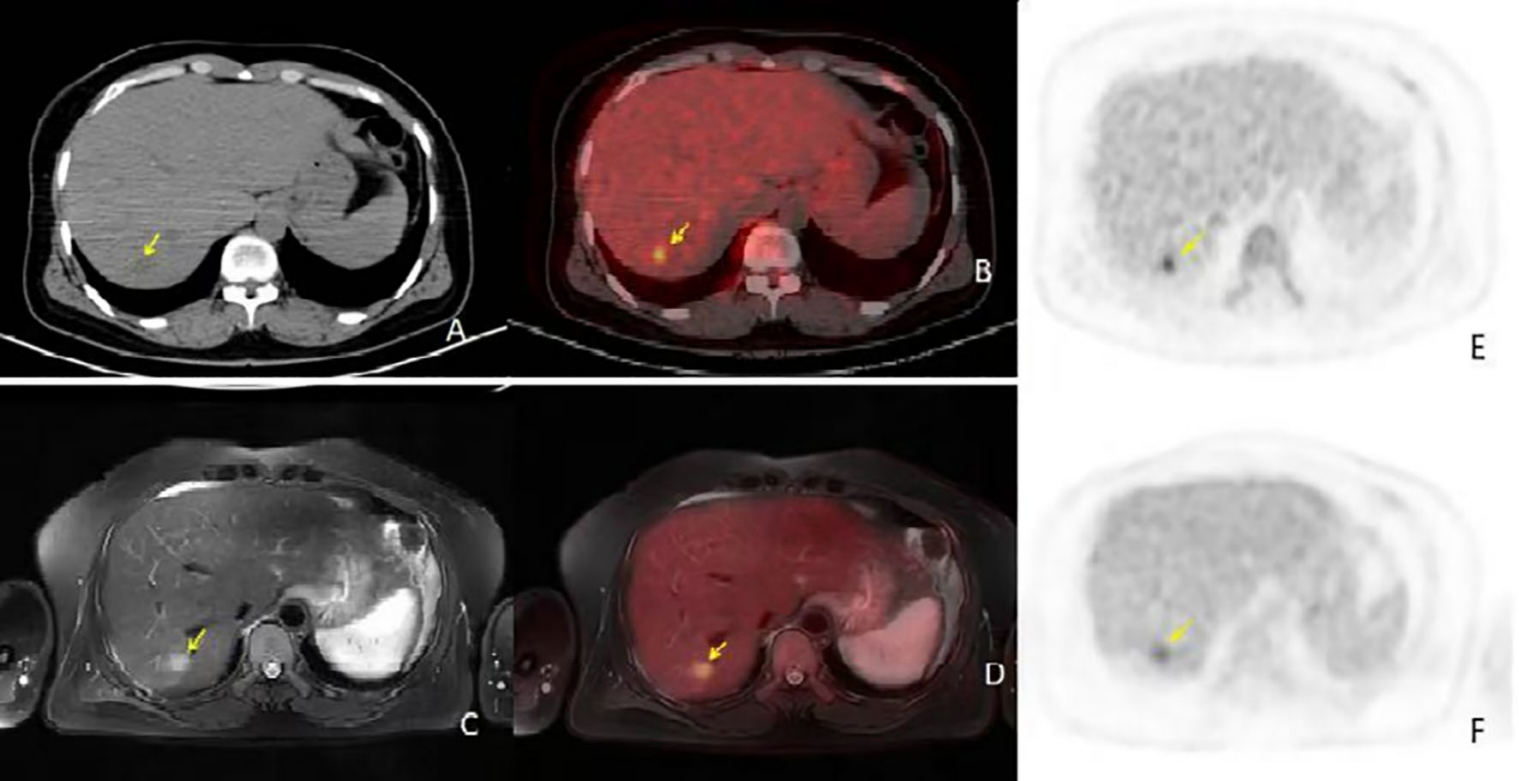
(A, B) are conventional PET/CT scan images [(A) is the CT image, (B) is the fusion image], (C, D) are PET/CT 2-hour delayed scan images [(C) is the CT image, (D) is the fusion image], and E and F are PET/MR images [(E) is the T2WI image, (F) is the fusion image]. The PET/CT lesion in the CT image shows isodensity, with no significant metabolic activity in the conventional scan. In the delayed scan, mild metabolic activity is seen, approaching the liver parenchyma, SUVmax 4.5. In the PET/MR image, the T2WI lesion shows high signal with mild metabolic activity, SUVmax 4.0. PET/MR provided a clearer diagnosis compared to PET/CT, and the PET/CT 2-hour delayed imaging offered a clearer diagnosis compared to conventional imaging.
